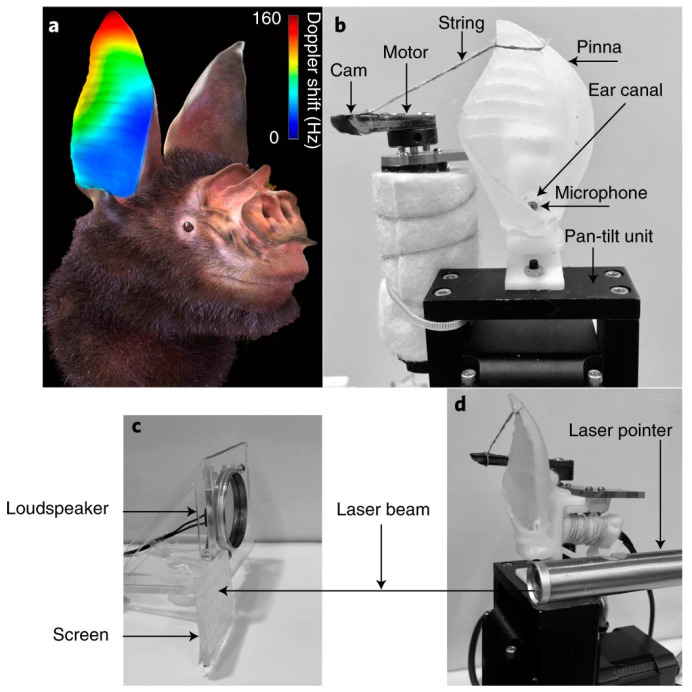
Acoustic and vibration sensing imitation with a bat-inspired ear structure. (a) Complex Doppler-shift distribution generated by dynamic deformation of the real pinna surface of Pratt’s roundleaf bat; (b) Silicone-based biomimetic robotic pinna and its experimental setup; (c) Frequency-generating speaker and alignment screen used in the experiment; (d) Biomimetic pinna equipped with a laser pointer to verify its orientation (adapted from [101]).
